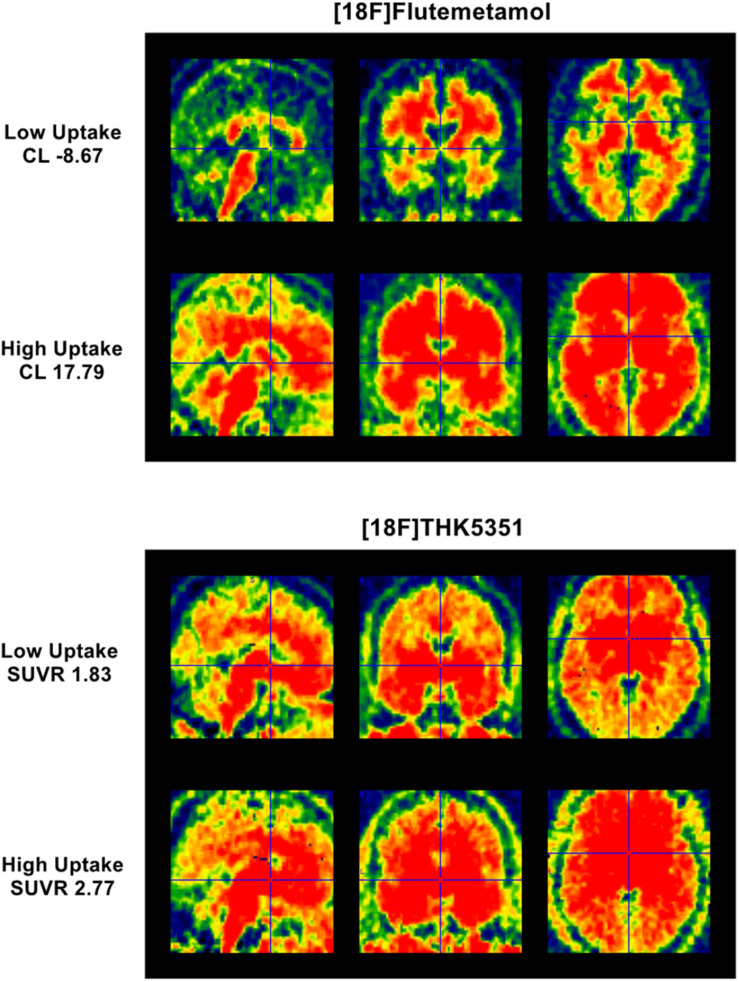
Parametric [18F]Flutemetamol and [18F]THK5351 uptake. (Top) panel represents parametric [18F]Flutemetamol binding estimation for subject having lowest Centiloid value (–8.67 CL) and for subject having highest Centiloid value (17.79 CL) over a mask covering mostly neocortical regions. (Bottom) panel represents parametric [18F]THK5351 binding estimation for subject having lowest SUVR value (1.83 SUVR) and for subject having highest SUVR value (2.77 SUVR) over a mask covering entorhinal cortex and hippocampus.
